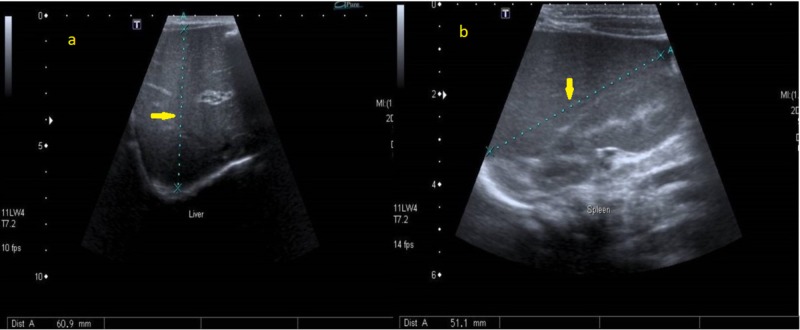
Abdominal ultrasound of the patient showed hepatosplenomegaly (a) Liver, (b) Spleen.
